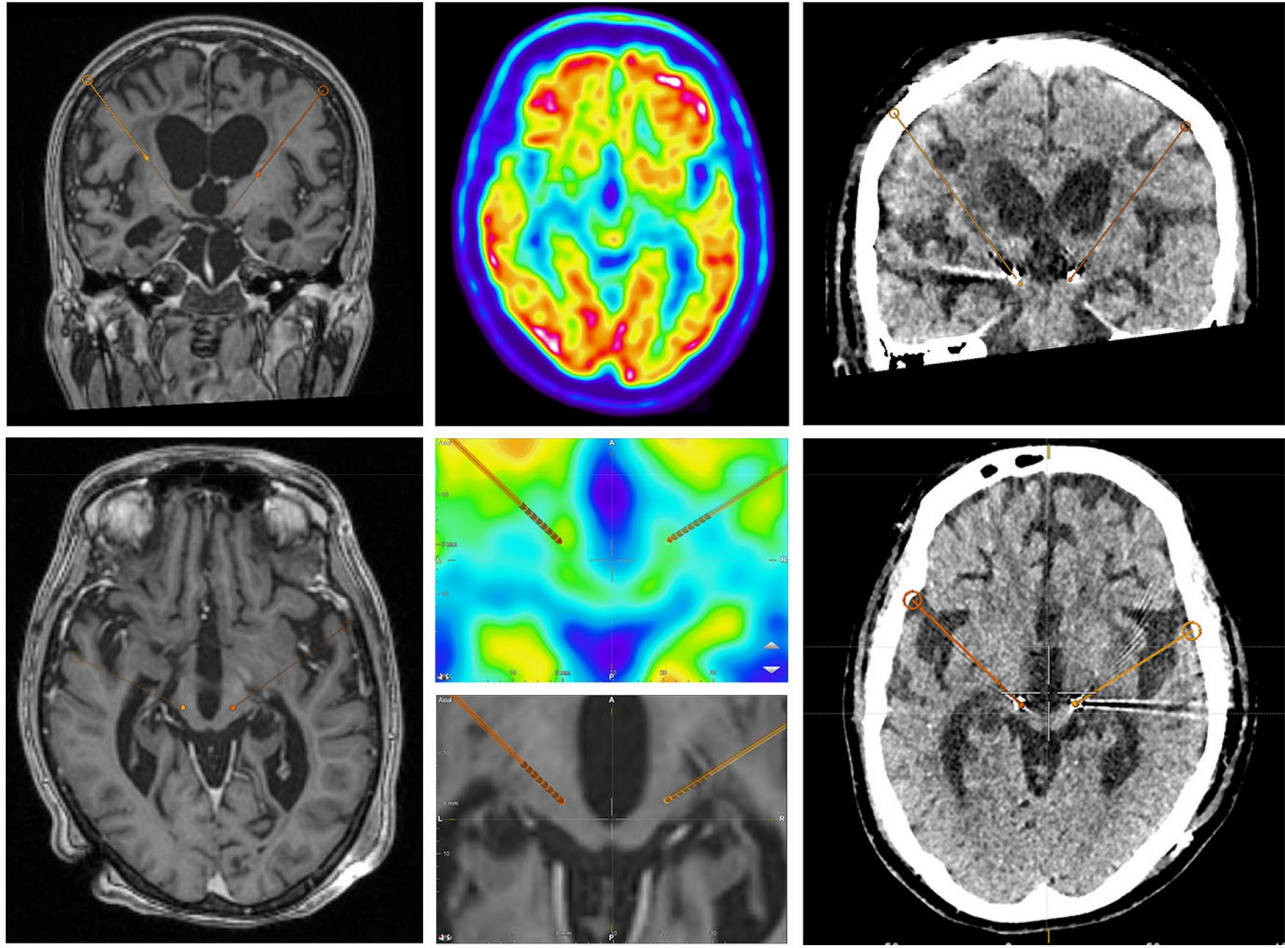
Left panel: intra-operative planning MRI with trajectories. Middle panel: ^18^F-FDG PET-scan of target areas, including planned trajectories. Right panel: post-operative CT-scan with electrode localization.
